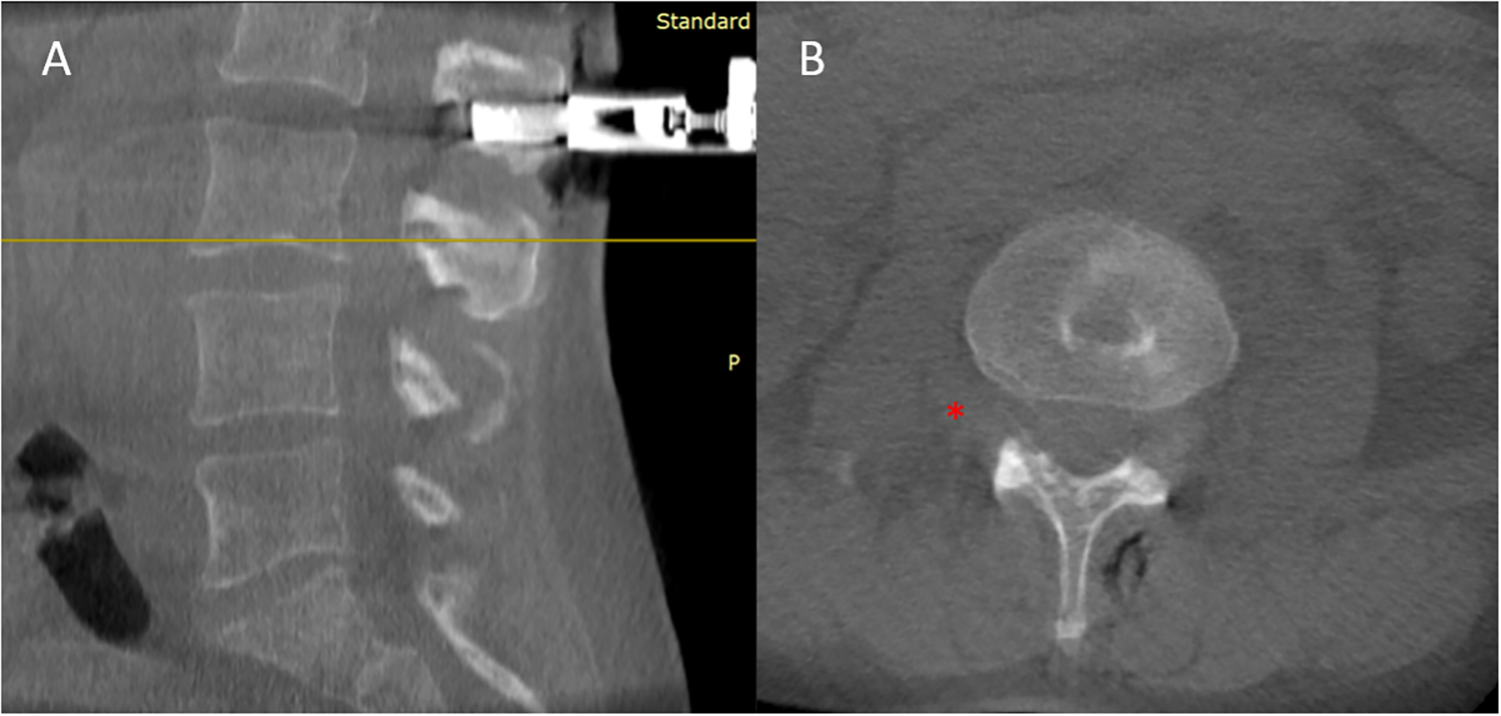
Intraoperative (A) sagittal and (B) axial CT-scan. The lesion (red asterisk) appears as a hypodensity lateral to the right L3–L4 foramen which extends beneath ipsilateral psoas muscle.
